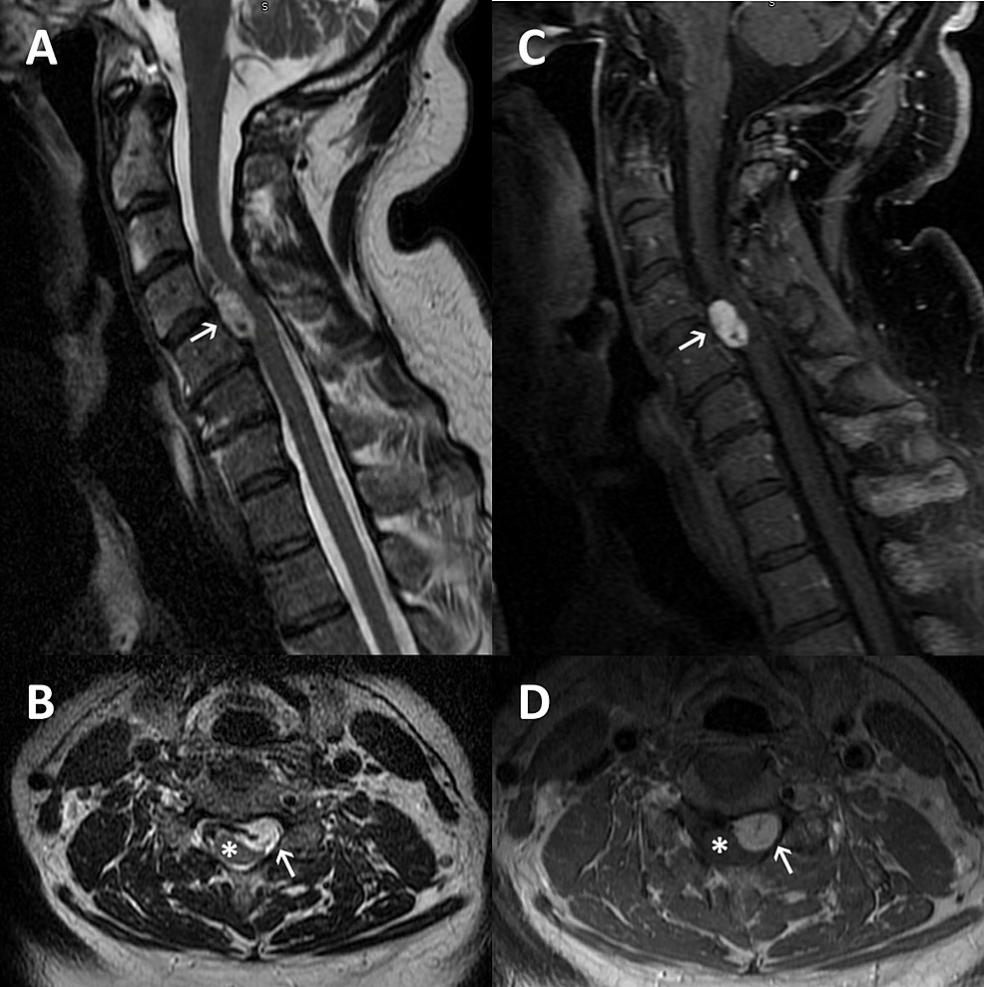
Pre-operative MRI MRI of the cervical spine demonstrating a left paracentral ventral intradural extramedullary lesion (white arrow) causing spinal cord (*) compression. (A) Sagittal view of a T2-weighted image; (B) axial view of a T2-weighted image; (C) sagittal view of a T1-weighted image with contrast administration; (D) axial view of a T1-weighted image with contrast administration.
